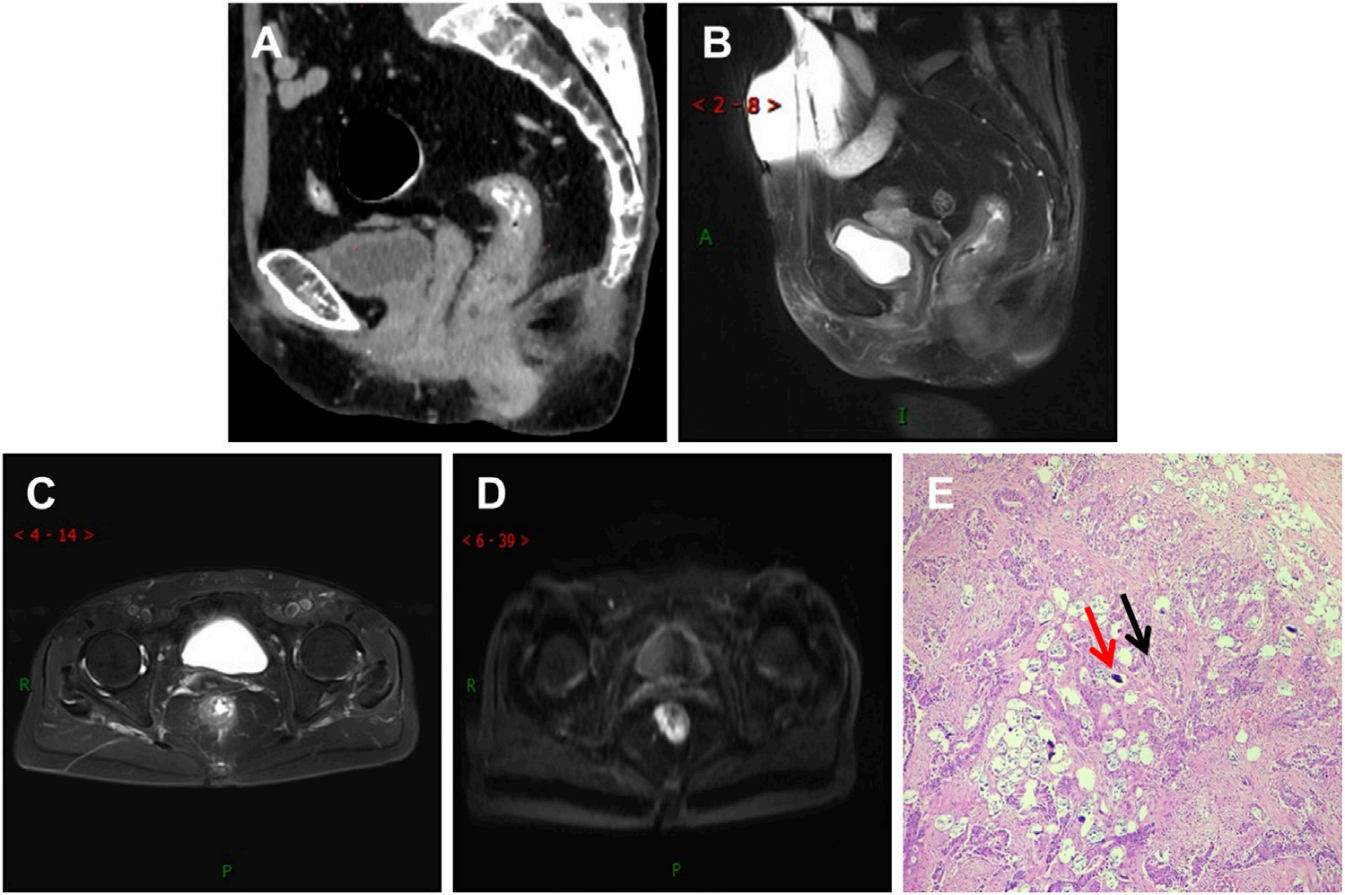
Differentiated adenocarcinoma of the rectum, where the tumor penetrates the intrinsic muscle layer to the exterior of the intestinal wall (T3N0MX stage). (A) CT shows significant thickening of the lower rectal wall and narrowing of the lumen, with multiple spotted and small strip-shaped calcifications visible within it; (B,C) T2WI sagittal and transverse images showed that the rectal tumor presented low signal intensity, thickening of the intestinal wall, rough edges, burry changes, incomplete muscularis mucosa lining, involvement of more than ½ circle of the intestinal cavity, and no lymph node shadow in the surrounding Mesentery fat space area; (D) The DWI sequence showed significantly high signal intensity in tumors; (E) In the pathological picture, the red arrow indicates Schistosoma eggs and the black arrow indicates tumor tissue. Schistosoma eggs are distributed in the tumor tissue.
